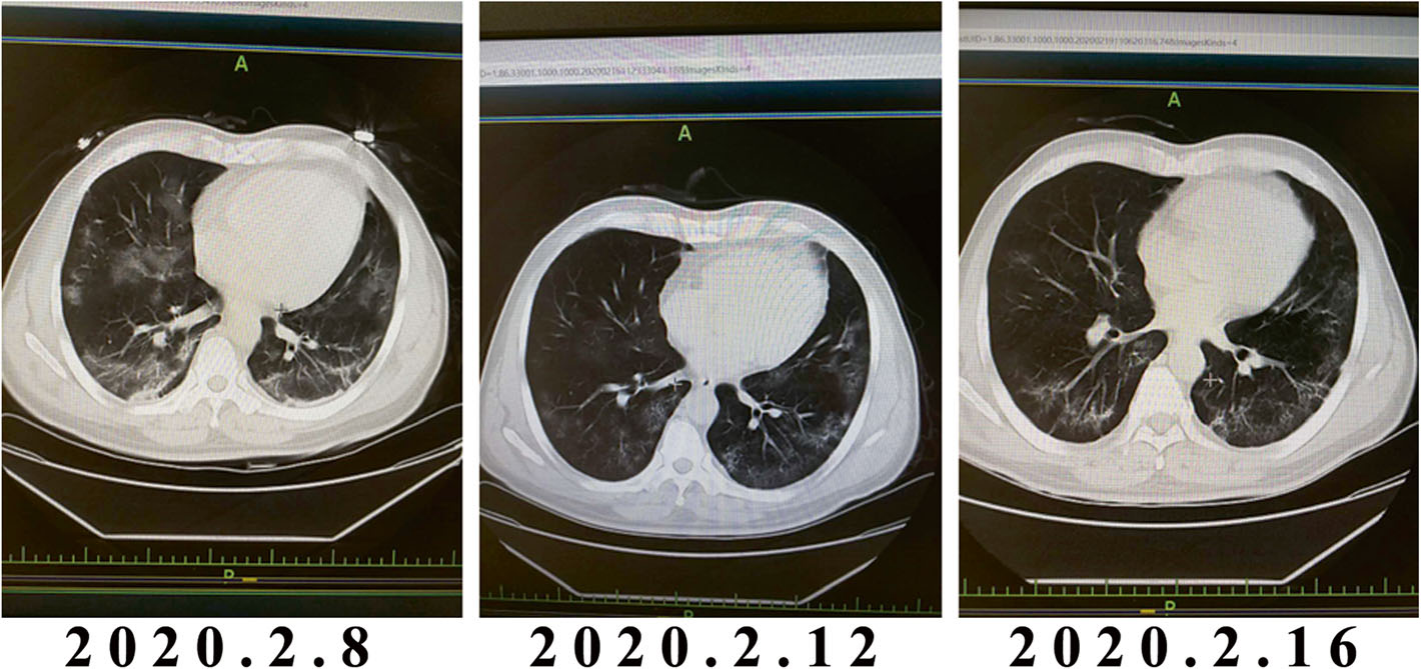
Thoracic CT images of the patient
